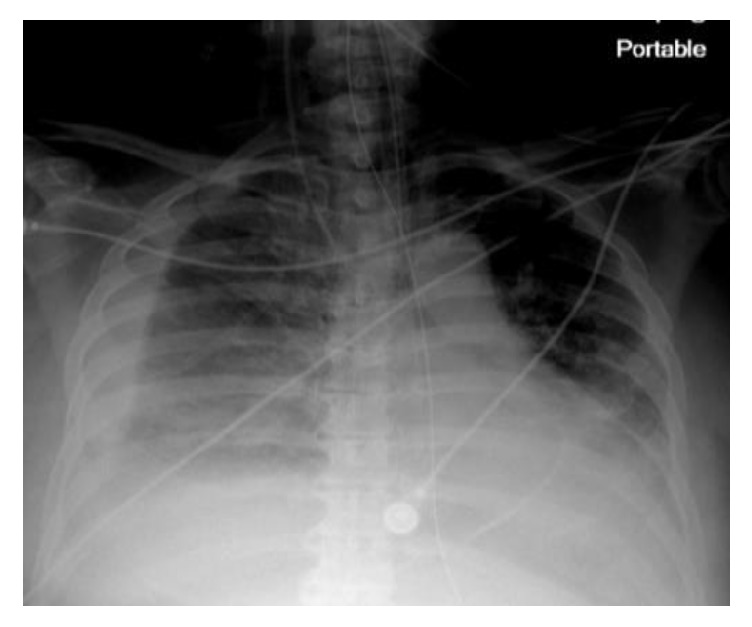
Post-op X-ray showing a foreign catheter tip in the heart.
